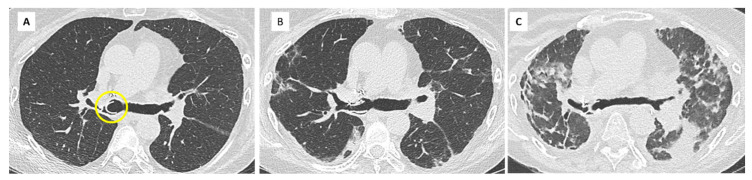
Acute exacerbation of RAS in a 67-year-old woman; (A) baseline CT scan. Stable obstructive pattern on PFT related to right main bronchus stenosis, treated by endo-bronchial stenting (yellow circle); (B) diagnosis of CLAD with restrictive profile. CT shows persistent subpleural opacities (ground glass mostly), consistent with RAS; (C) 1 month later, the patient presented with acute clinical and functional deterioration. CT shows extensive and rapidly progressive ground glass opacities, suggesting diffuse alveolar damage and/or AFOP. The patient died a few days later.
